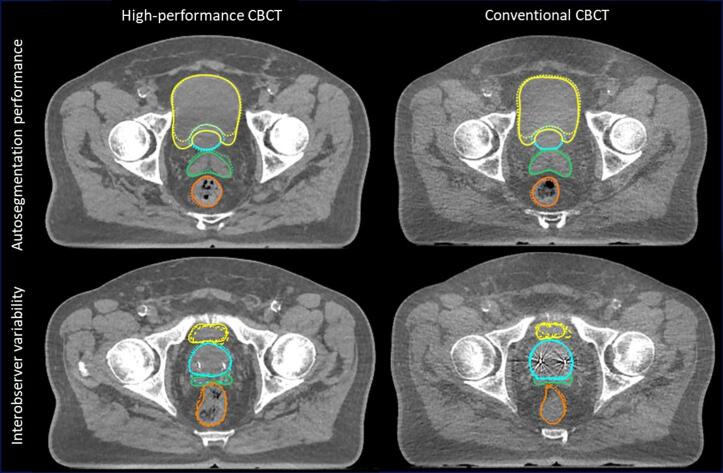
(color in print). Examples of CBCT image quality, autosegmentation performance and interobserver variability for a high-performance CBCT scan (left) and a conventional CBCT scan (right) of the same patient. The structures of the prostate (blue), seminal vesicles (green), bladder (yellow), and rectum (orange) are displayed. The bowel was also segmented, but is not depicted in these examples. Top row: Autosegmentation performance (dotted = artificial intelligence (AI)-based autosegmented contour, solid = fully corrected contour of one observer). Bottom row: interobserver variability (dot-dashed = observer 1, solid = observer 2, dashed = observer 3). Abbreviations: CBCT, Cone-beam computed tomography. (For interpretation of the references to color in this figure legend, the reader is referred to the web version of this article.)
